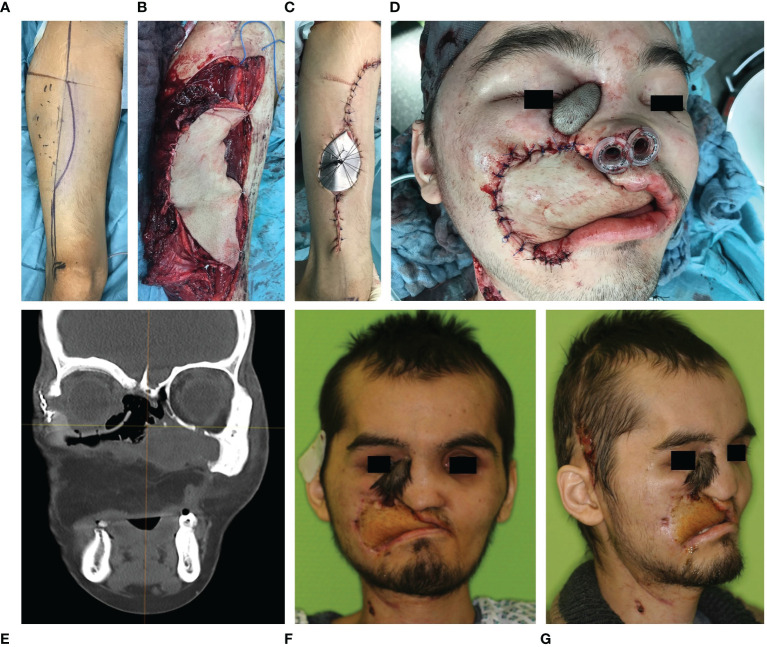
Early secondary soft tissue reconstruction of the upper lip and jaw using an anterolateral thigh flap in a case of Ewing’s sarcoma of the midface. (A–C) Skin marking, flap harvest and wound closure of the anterolateral thigh flap of the right lower extremity. (D) Anterolateral thigh flap sewed in. (E) Postoperative CT scan in coronal plane shows the patient specific implant *in situ* reconstructing the right orbital floor while the symmetrical position of the eyeball has been restored. (F) Frontal and (G) half site view of the patient two months after tumor resection and two stage reconstruction (primary orbital floor and early secondary soft tissue of the upper lip and jaw).
